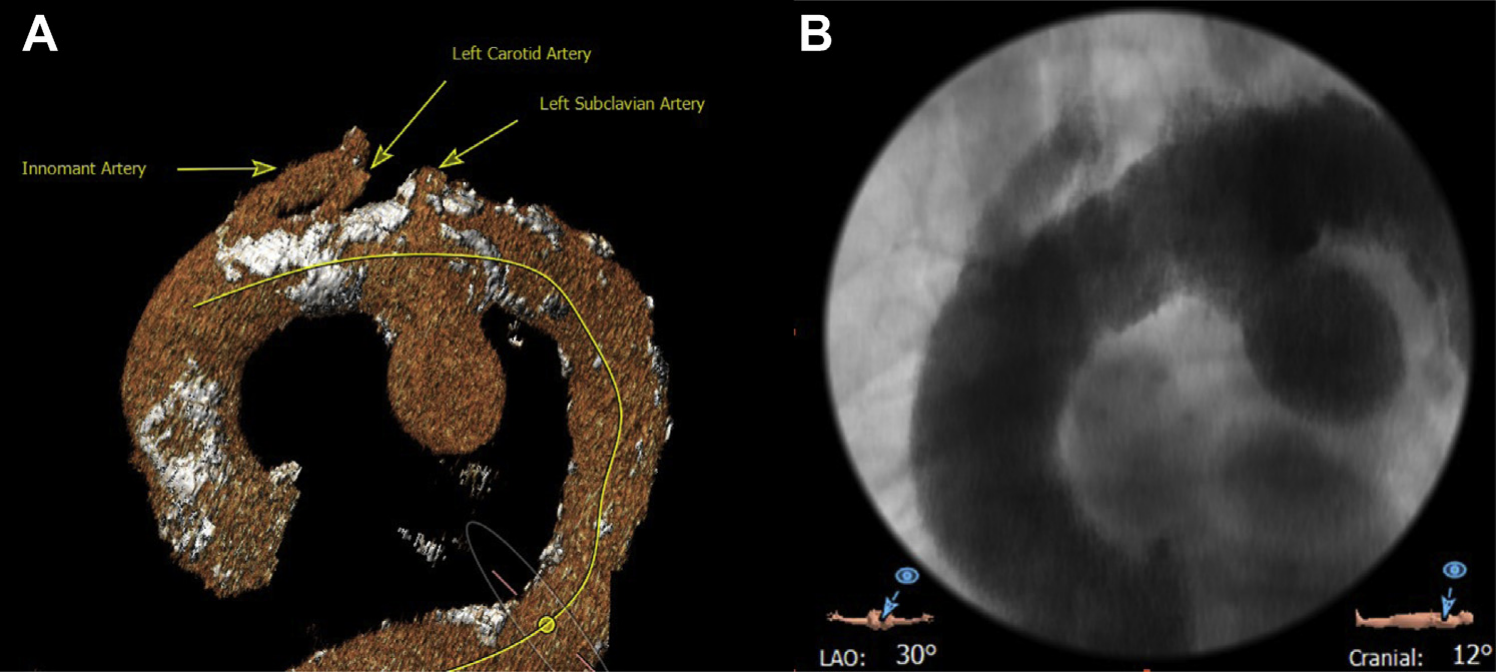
A, Computed tomography three-dimensional reconstruction showing a saccular thoracic aneurysm in relation to the aortic arch vessel anatomy. B, Intraoperative angiogram showing saccular thoracic aneurysm filling with contrast.
